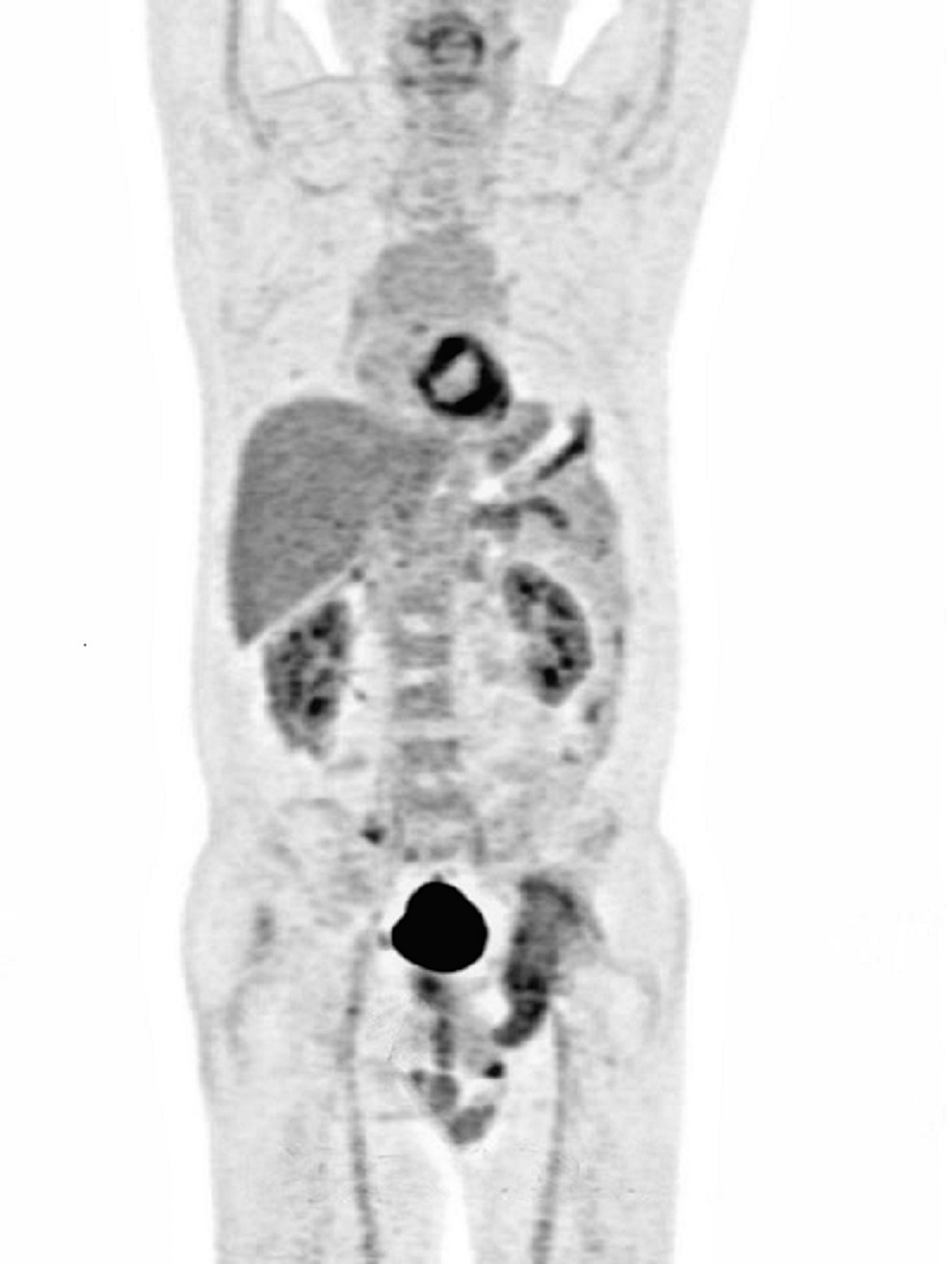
F18 FDG whole-body PET-CT Radiological carcinoma of the prostate with bone and lung metastases.
